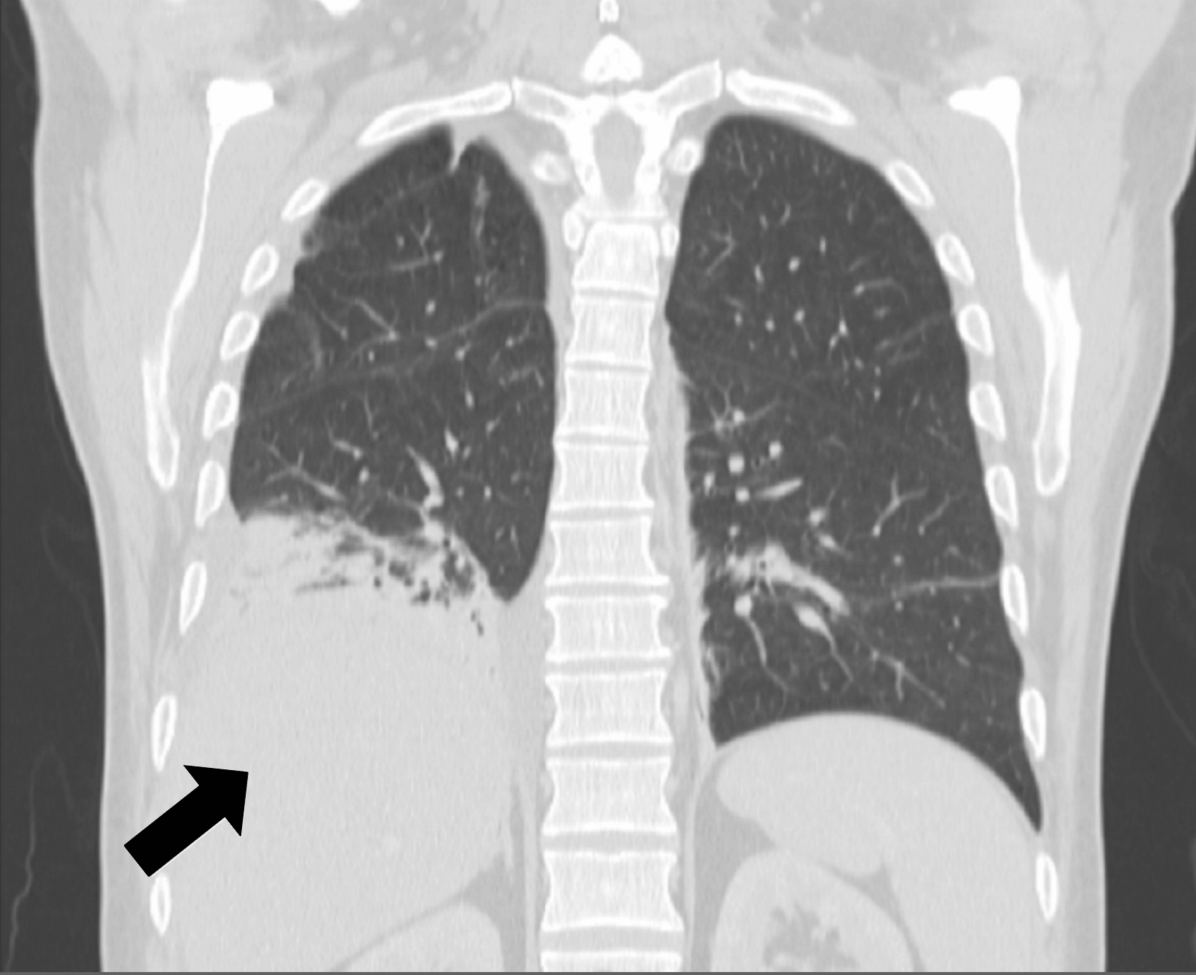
Chest CT on the 8th day of hospitalization, coronal view. Chest CT on the 8th day of hospitalization, corresponding to the patient's clinical deterioration, showing new consolidation in the right lung.
